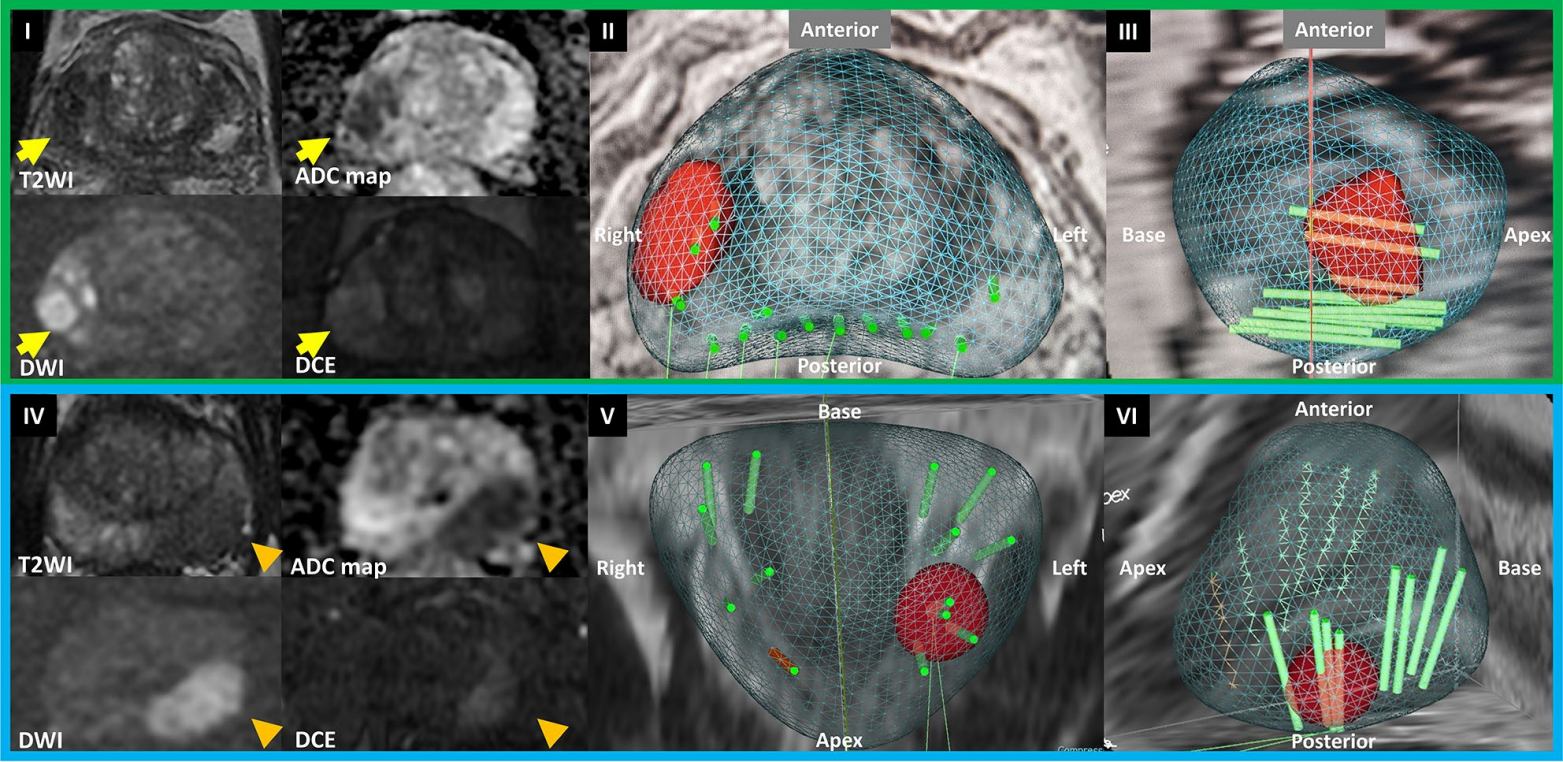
Representative images of transperineal and transrectal MRI/TRUS fusion prostate biopsy. (I–III) Transperineal MRI/TRUS fusion prostate biopsy (I) Pre biopsy mpMRI showing PIRADS score 5 lesion (yellow arrow) in the right mid peripheral zone. (II) Axial and (III) right sagittal view of Transperineal MRI/TRUS fusion prostate biopsy. (IV-VI) Transrectal MRI/TRUS fusion prostate biopsy. (IV) Pre biopsy mpMRI showing PIRADS score 5 lesion (orange arrowhead) in the left mid peripheral zone; (V) coronal view and (VI) left sagittal view of Transrectal MRI/TRUS fusion prostate biopsy. *MRI* magnetic resonance imaging, *TRUS* transrectal ultrasound, *mp* multiparametric, *PIRADS* prostate imaging reporting & data system, *T2WI*, *T2* weighted image, *ADC* apparent diffusion coefficient, *DWI* diffusion-weighted imaging, *DCE* dynamic contrast-enhanced.
